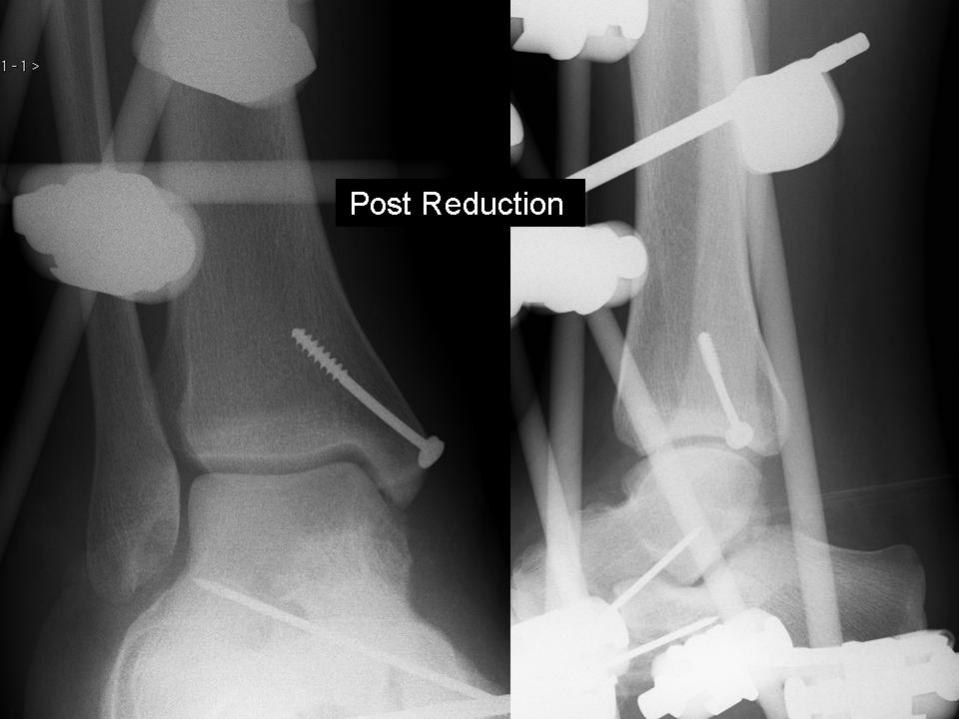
Anterio-posterior and lateral radiograph post reduction.
